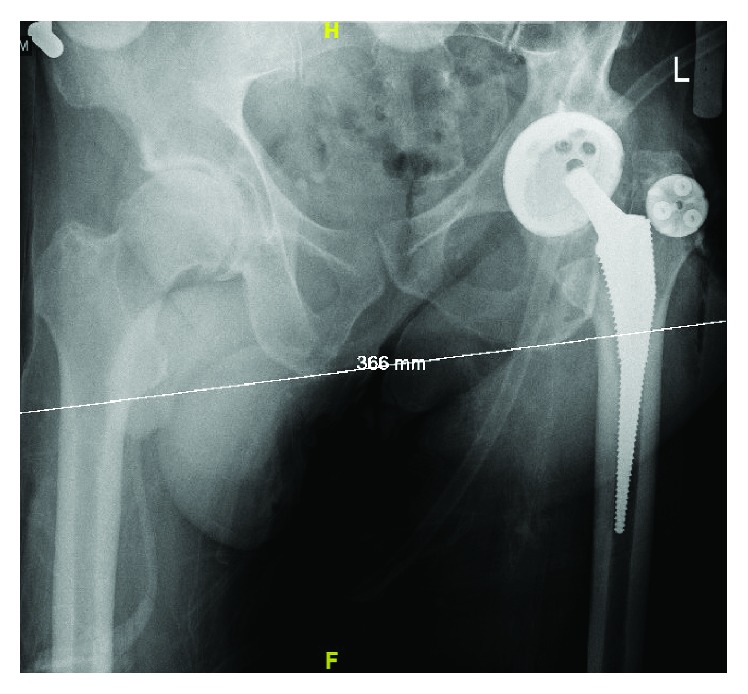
Intraoperative X-ray of the left hip demonstrating satisfactory positioning of the implants. The tracker base for the camera is visualized on the greater trochanter of the femur.
